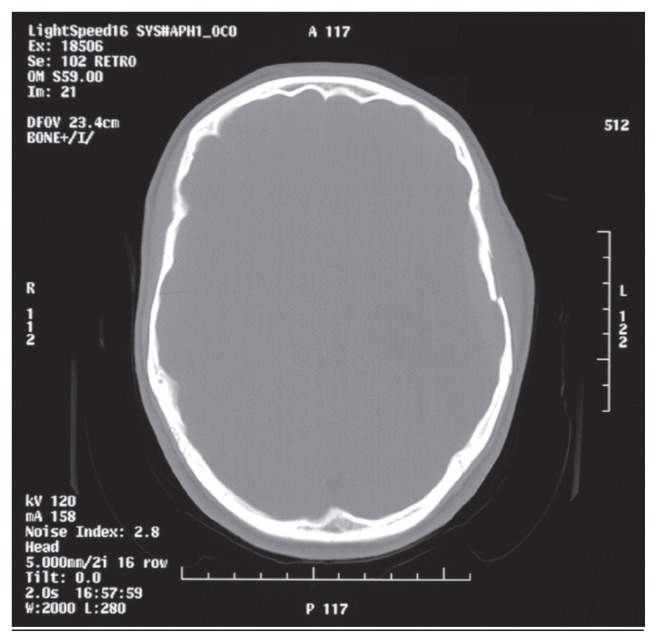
CT scan (bone window) demonstrating the temporal bone fracture.
